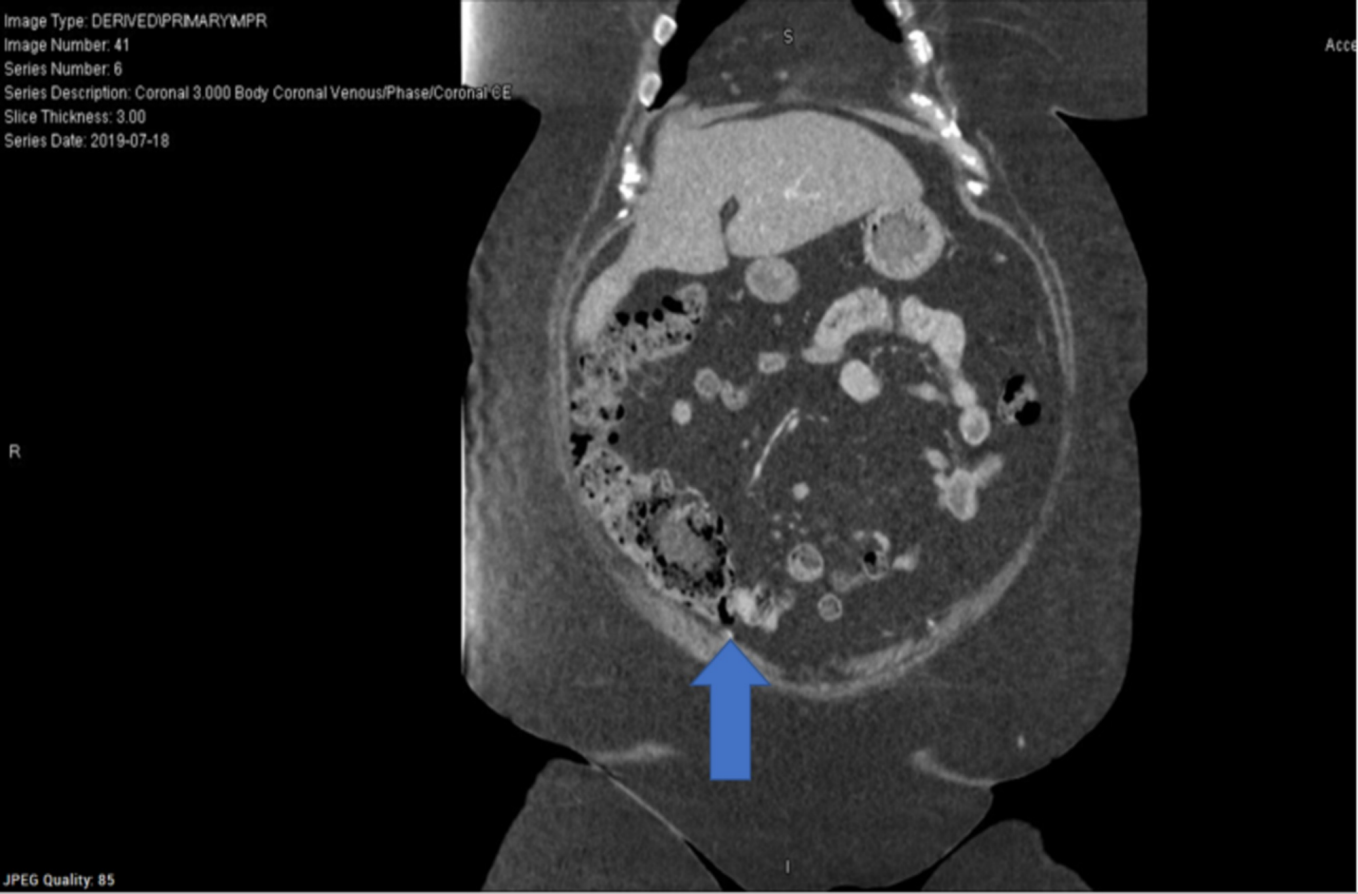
CT abdomen - Thickened appendix with possible mild inflammation. Acute appendicitis is suspected. - Umbilical hernia containing fat. CT: computed tomography
